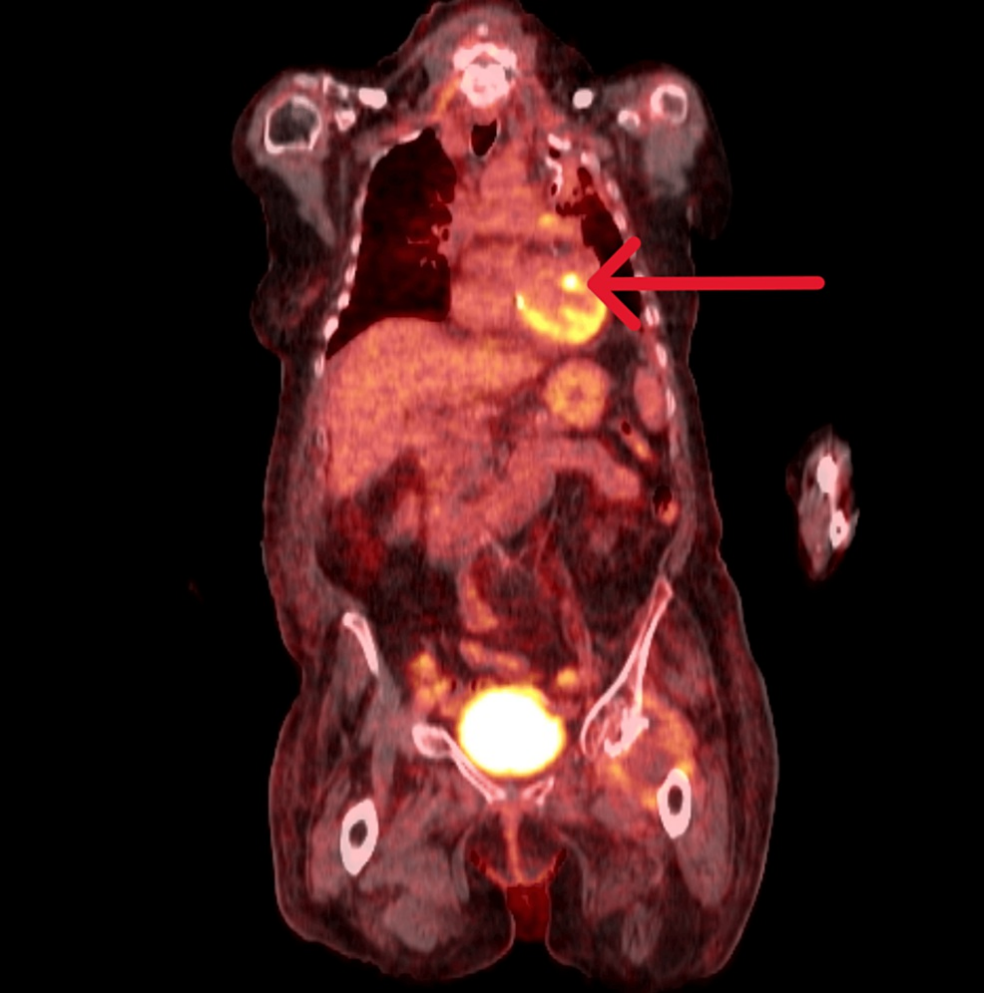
Coronal section of 18F-FDG PET/CT showing increased FDG uptake of mitral valve 18F-FDG, fluorine-18-fluorodeoxyglucose; FDG, fluorodeoxyglucose
